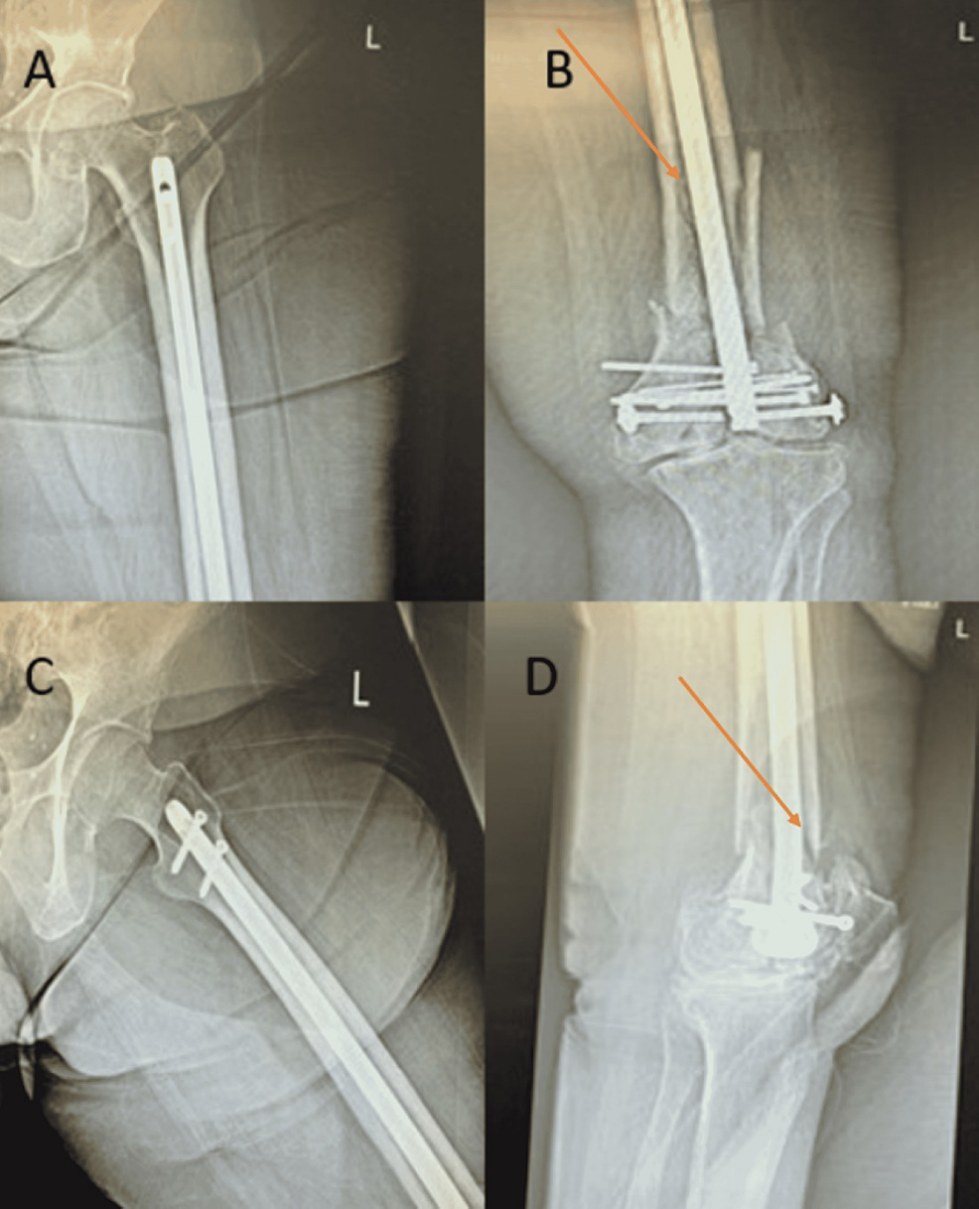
Three-month postoperative imaging revealing hardware failure of distal femur fracture. A: AP X-ray of the left hip. B: AP X-ray of the left knee. C: Frog-leg X-ray of the left hip. D: Lateral X-ray of the left knee. Arrows indicate a lack of osseous formation.
